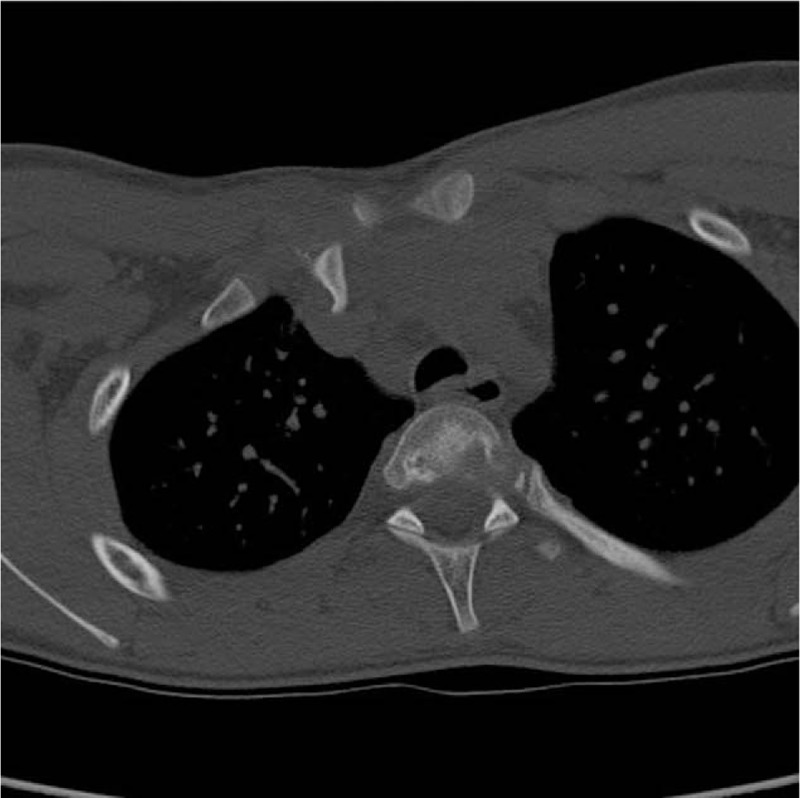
Computed tomography scan showing a right posterior sternoclavicular joint pseudodislocation.
